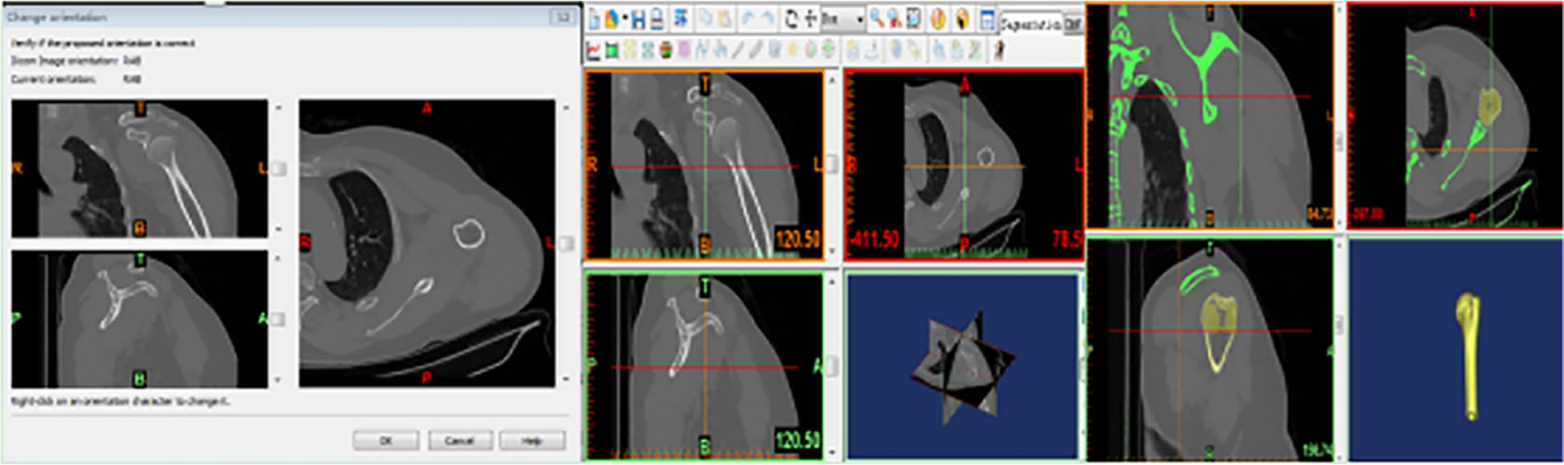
CT image data was imported into Mimics 17.0 software. Construct a 3D model of the humerus
